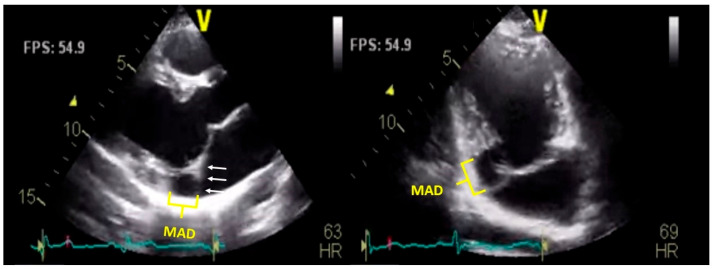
Transthoracic echocardiographic images demonstrating mitral annular disjunction (MAD). Parasternal long-axis view (left) and apical four-chamber view (right) reveal a distinct separation between the posterior mitral annulus (white arrows) and the basal inferolateral left ventricular myocardium (yellow brackets). The arrows highlight the disjunction plane in systole. MAD is visualized as a systolic detachment of the mitral annulus from the ventricular wall, most prominently affecting the posterior annulus.
